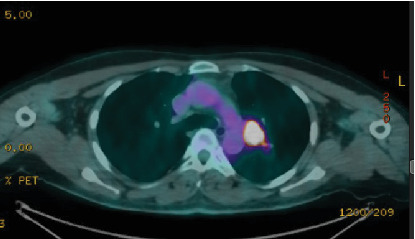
Whole-body PET scan showing FDG-avid left hilar lymphadenopathy.
